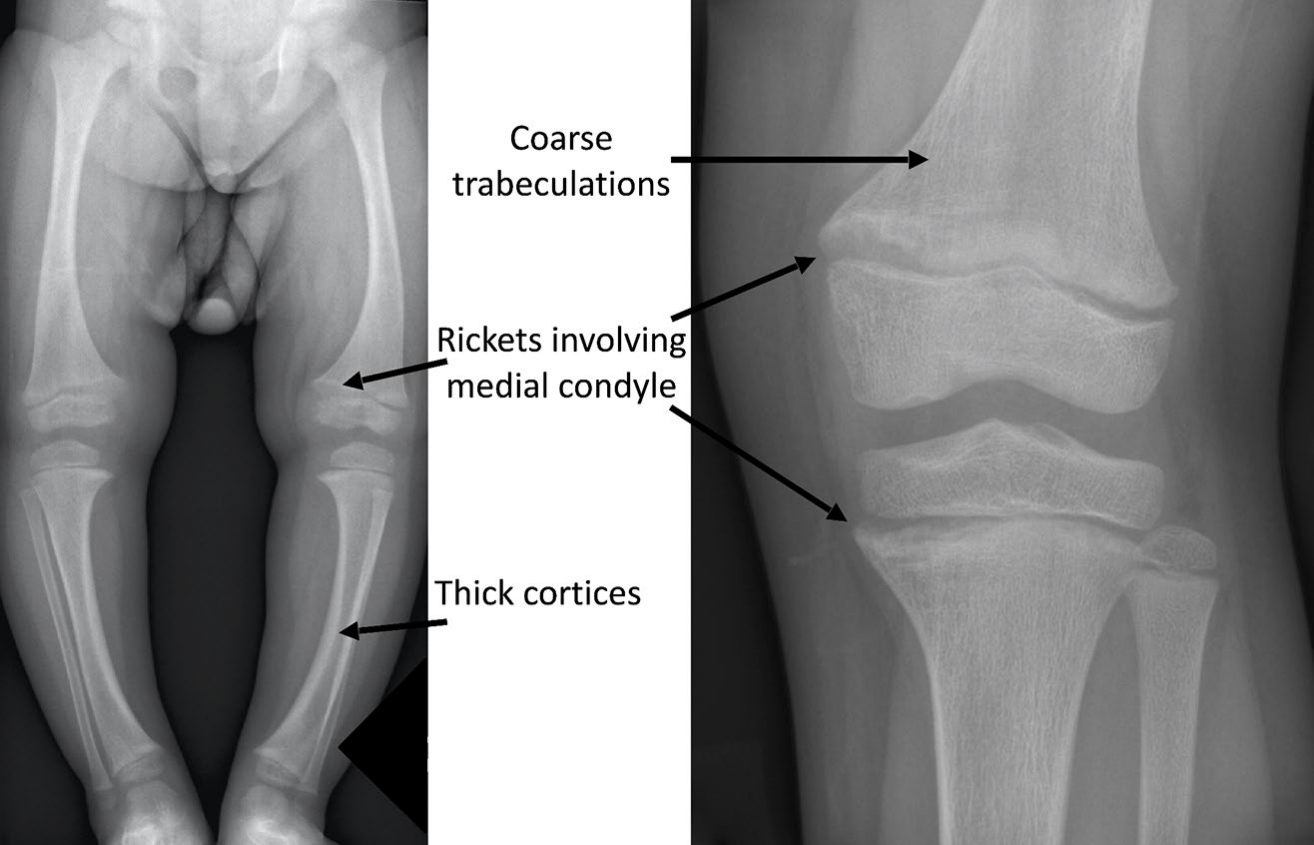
Radiological features of X-linked hypophosphatemic rickets. Lower limb and knee radiograph of a 5-y-old female showing characteristic changes of rickets affecting one of the femoral and tibial condyles, coarse trabeculations of the bones and thick cortices
